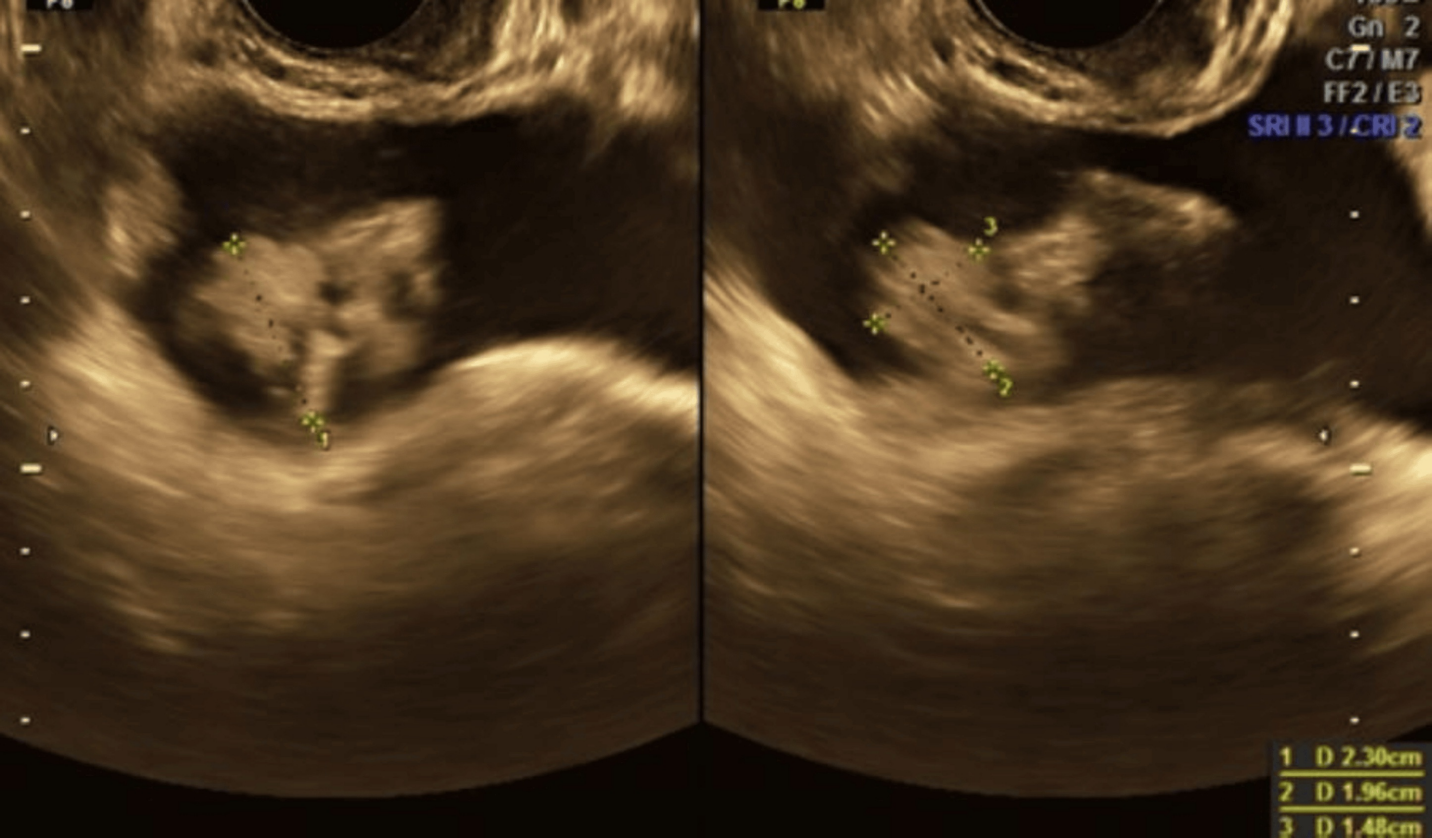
Ultrasonography showing a small echogenic polypoidal lesion measuring 27 × 17 mm along the anterolateral wall of the right side of the urinary bladder.
